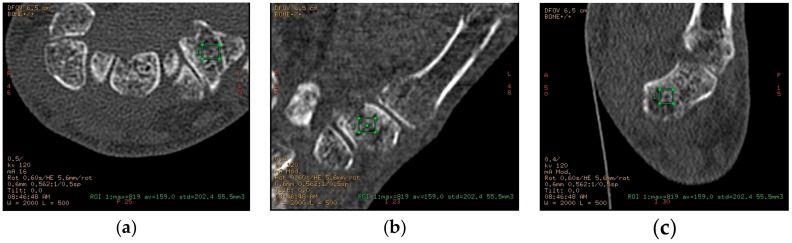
(a) Bone mineral density determination by measuring CT attenuation (in HU) in a three-dimensional volume, defined by a rectangular ROI in axial plane; (b) Bone mineral density determination by measuring CT attenuation (in HU) in a three-dimensional volume, defined by a rectangular ROI in coronal plane; (c) Bone mineral density determination by measuring CT attenuation (in HU) in a three-dimensional volume, defined by a rectangular ROI in sagittal plane. Green square: The region of interest on CT scan which was placed in the cancellous bone of the trapezium in all three (a, b, c) scan planes for assessment of HU values.
